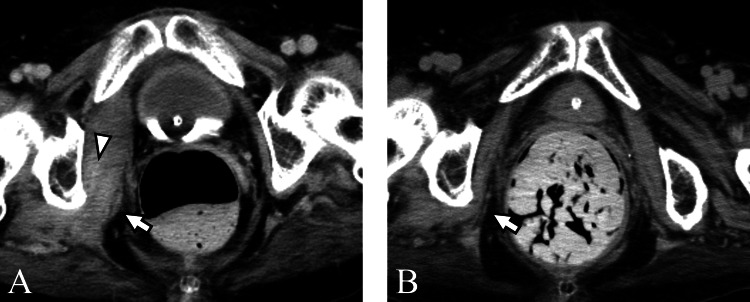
Case three A 69-year-old woman underwent a CT to evaluate fever source. (A) CT on the 18th day of hospitalization revealed swelling (arrow) and calcification (arrowhead) in the right muscles. Manual muscle testing score in the right lower limb was 0. Deep vein thrombosis was the suspected source of the fever. (B) On the 103rd day, the calcification disappeared, and no ossification was observed (arrow).
